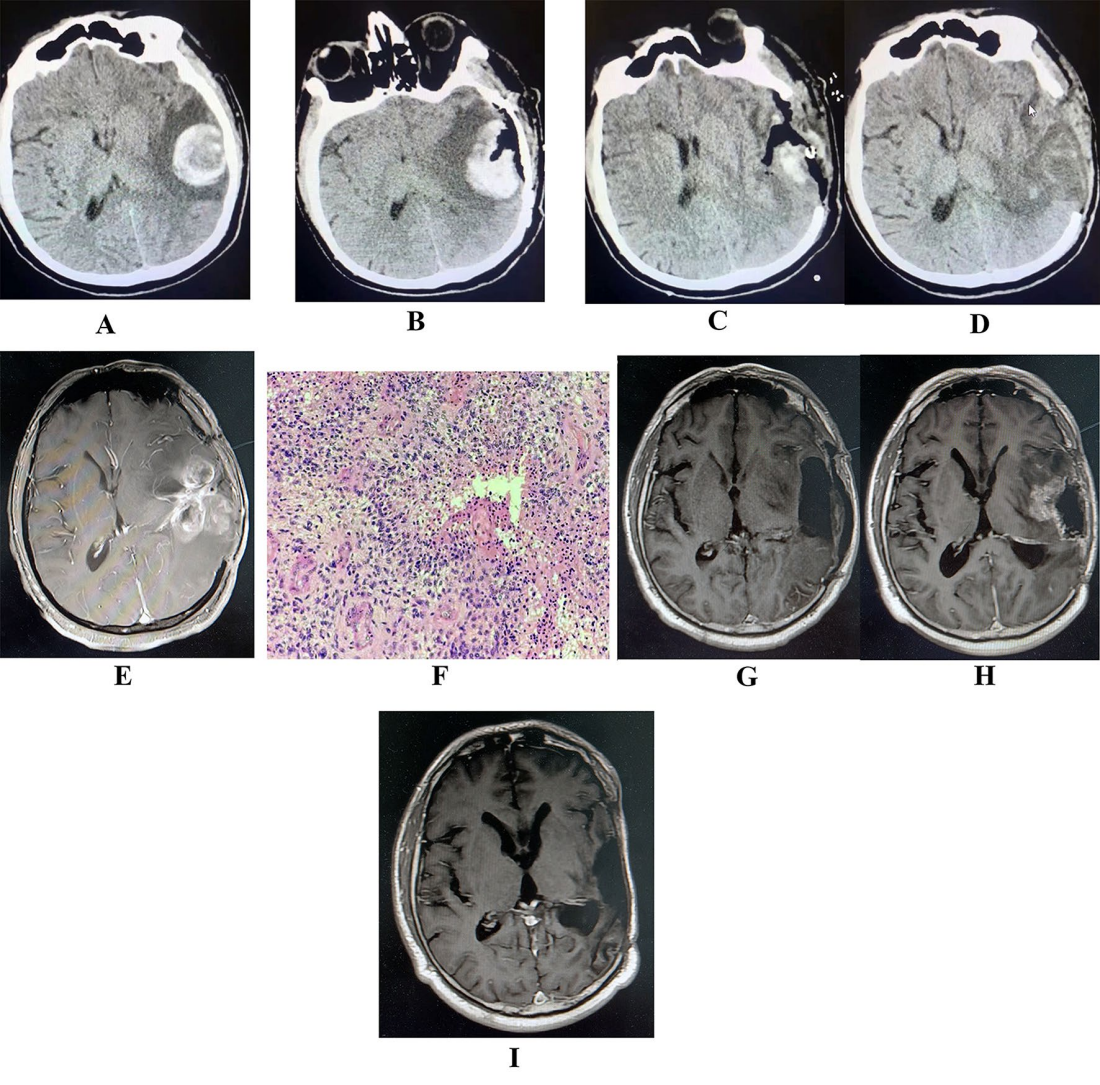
A. Head CT performed two hours after the onset of symptoms reveals a left temporoparietal hemorrhage with perihematomal edema. B: Twelve hours after the craniotomy hematoma evacuation, the patient remained unconscious, and a follow-up head CT shows a hemorrhage in the operative area, while the brain tissue in the edema area remains unchanged. C: Subsequent head CT after a secondary craniotomy shows a clean evacuation of the hematoma. D: Twenty days post-surgery, the head CT indicates the emergence of cerebral edema in the left temporoparietal lobe. E: A cranial enhanced MRI reveals a space-occupying lesion in the left temporoparietal lobe, indicating a potential high-grade glioma. F: The pathological analysis indicates a glioblastoma of WHO-grade 4. G: Following the surgical removal of the tumor, a review of the head MRI confirms a complete resection without any residue. H: Four months after the glioblastoma resection, a follow-up cranial MRI shows in-situ tumor recurrence. I: A cranial MRI, taken after four months of concomitant temozolomide and bevacizumab treatment, shows the disappearance of the primary recurrent glioma
